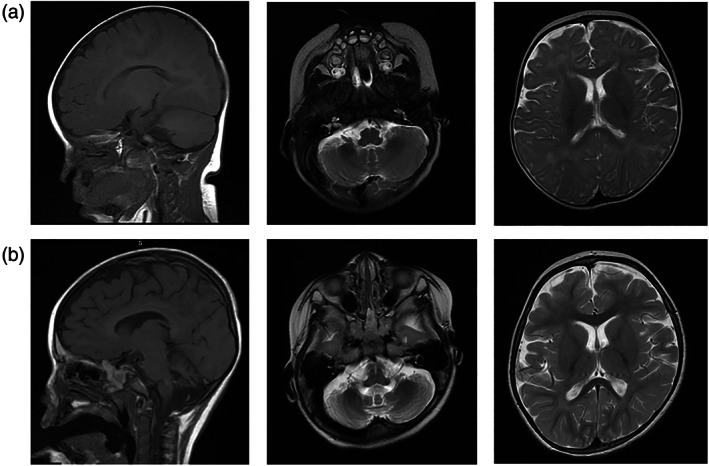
Magnetic resonance imaging for child with biallelic CACNA1A variants. (a) Cranial MRI at 6 months of age. (b) Cranial MRI at 3 years of age. Both panels show T1‐weighted sagittal image and T2‐weighted axial images
